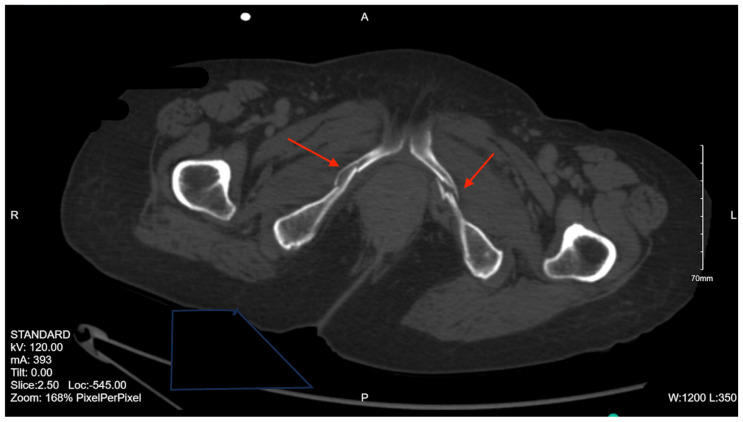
CT abdomen/pelvis images of the described fractures in case 9. Red arrows are used to indicate the location of pelvic fractures for easier identification.
